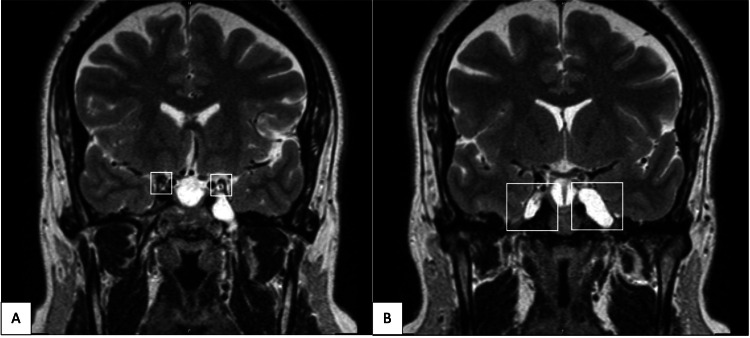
Coronal T2 brain MRI showing signs of intracranial hypertension. A: Dilated and fluid-filled oculomotor cisterns. B: Bilateral enlarged Meckel’s cave and bilateral tortuosity of optic nerves with increased CSF spaces.
